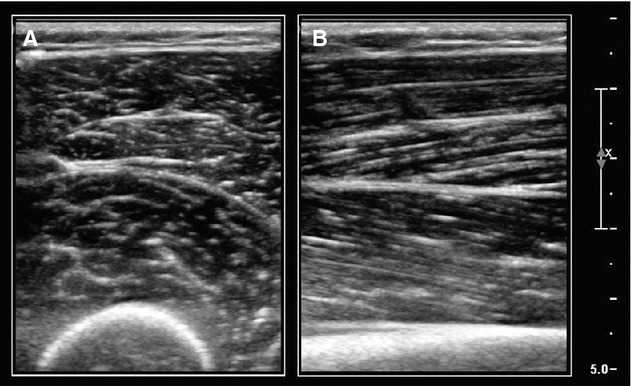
Muscle fascicle orientation in the mid thigh visualized using B-mode ultrasound. (A) coronal plane, perpendicular to long axis of leg as used for CEUS recordings, (B) sagittal plane view of the same region demonstrates *penate* nature of quadriceps muscles; fascicles are orientated at an angle to the long axis of the muscle.
